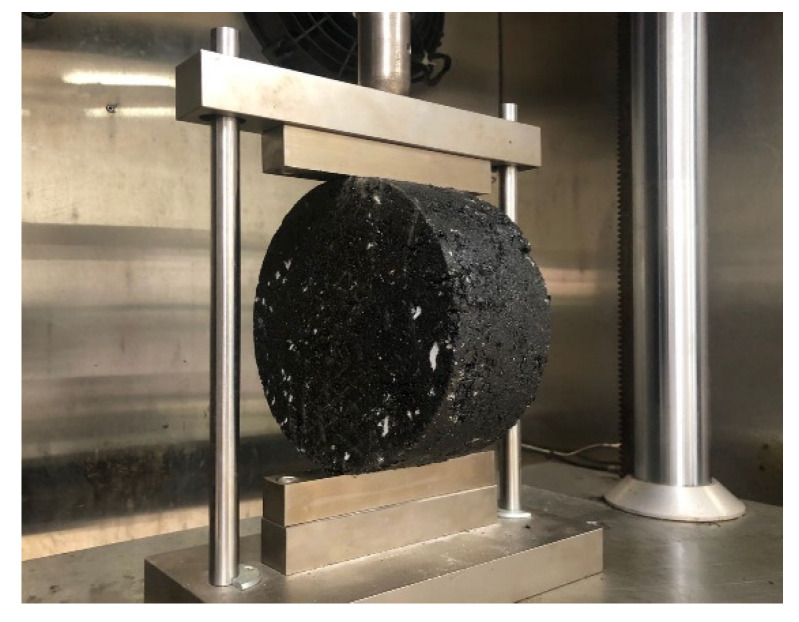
IDEAL cracking test (IDEAL-CT).
